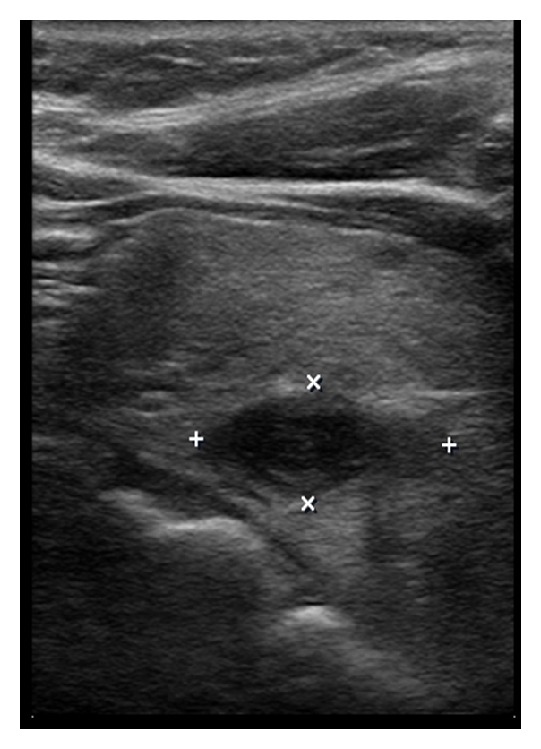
Ultrasound number 2 of left lower parathyroid gland—8/9/12: after infarction.
